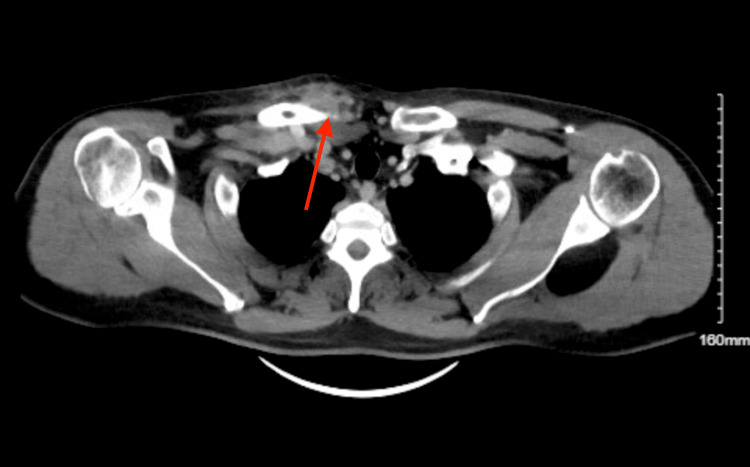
CT chest with IV contrast, transverse view: further demonstrates the same findings.
